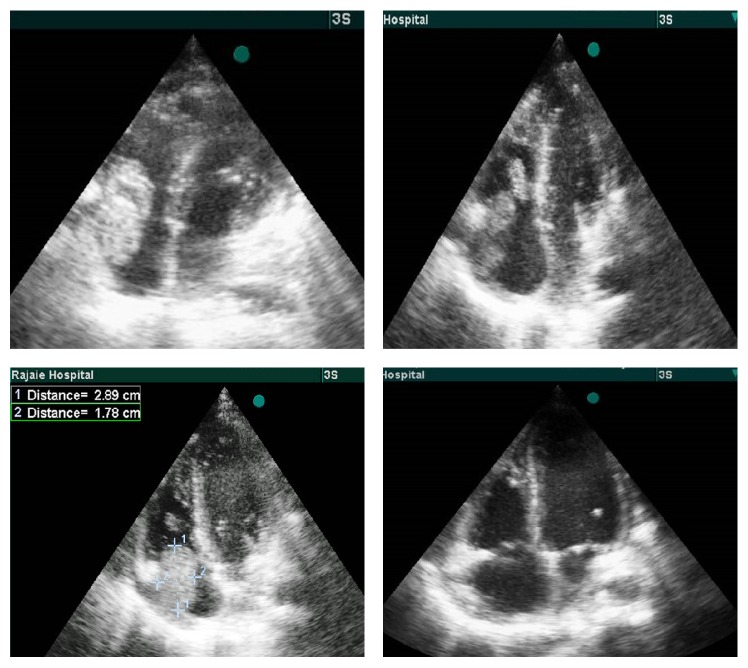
Right atrium vegetation in different view in echocardiography subcostal; four chamber views during systole and diastole. Note mobile position of mass that enters RV during diastole. Last image showed four chamber views after mass resection.
